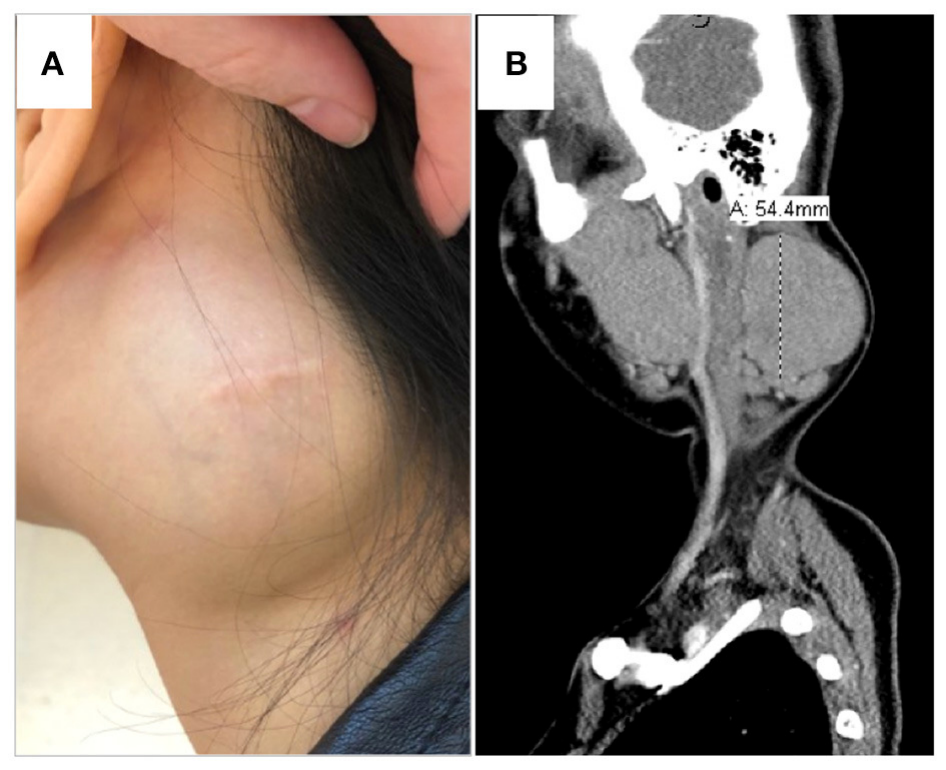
Left neck mass. (A) Physical exam displayed a prominent, firm left neck mass, roughly 5 × 5 cm, which was immobile, non-tender, non-fluctuant and marked by a healed surgical scar. She had an additional small, healed, post-auricular surgical scar. (B) CT scan demonstrated a 5.4 × 3.7 × 6.3 cm, well-delineated, non-calcified, homogenous, non-necrotic nodal mass.
